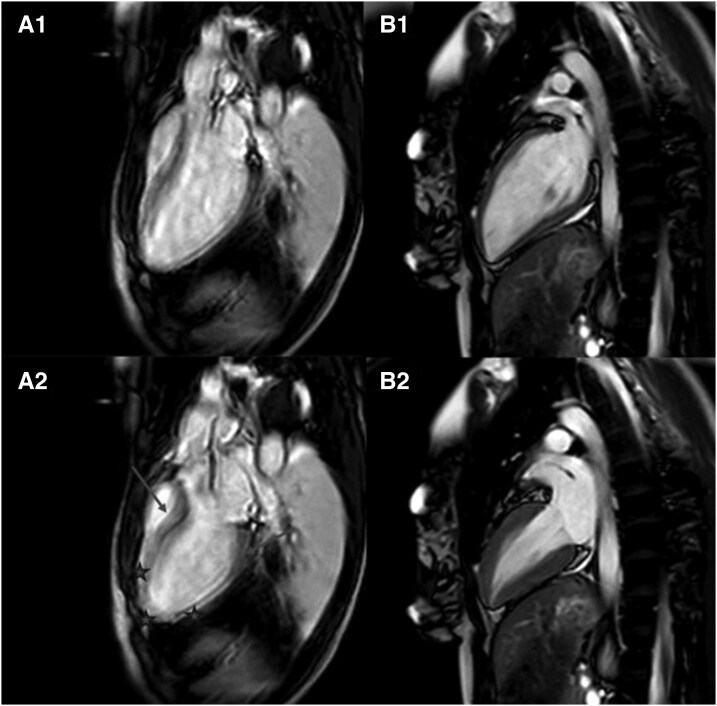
Cardiovascular magnetic resonance images demonstrate the apical ballooning in the acute phase (A1, A2), whereas in the control examination (B1, B2) a restoration of left ventricular function and ventricle geometry can be observed.
